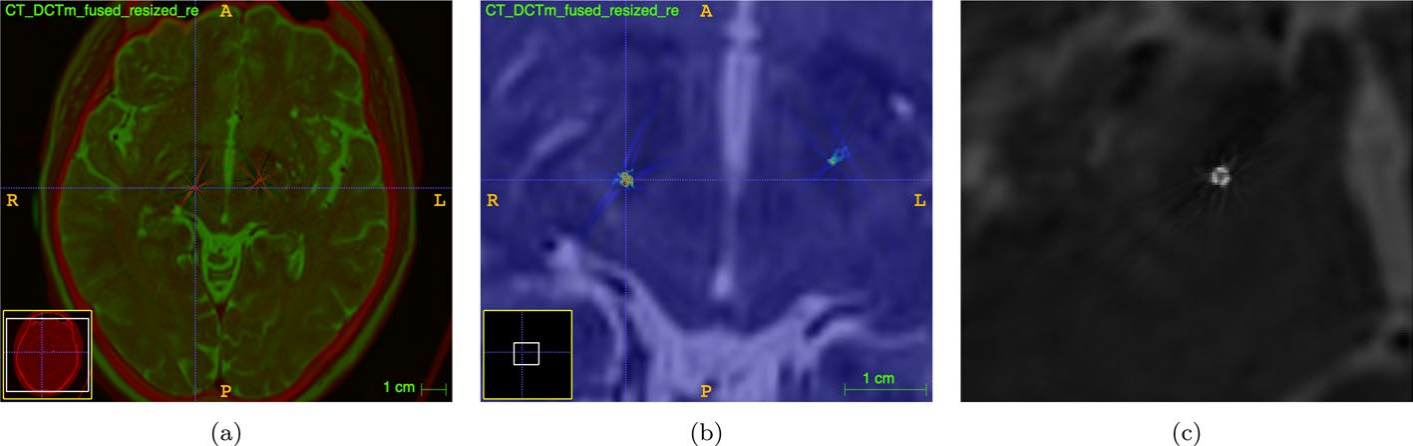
Demonstration of registration to MRI as possible postprocessing-step in future work (mean TRE: 1.7 mm, STD: 0.93 mm using ITK-SNAP Yushkevich et al. 2006), a CT is displayed in red and T2-weighted MRI in green, b segmented contacts of right electrode in red according to a hsv color-map, c after multi-planar-reconstruction (MPR) along an electrode based normal vector: the three segmented contacts can now be clearly delimited in grayscale color map
